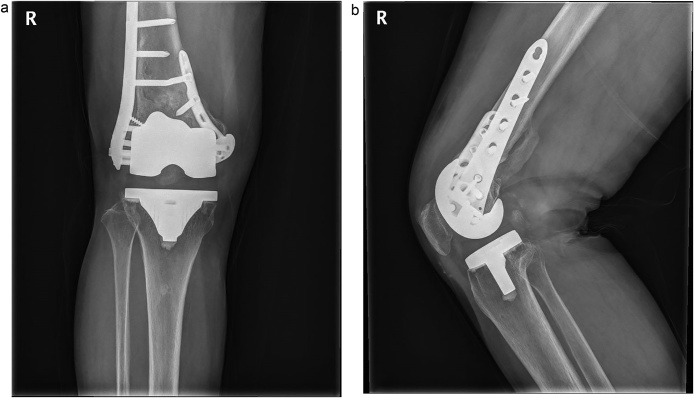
In the postoperative 6 months, radiographs show complete fracture healing after dual plating on both sides. (A) anterioposterior radiograph (B) lateral radiograph.
